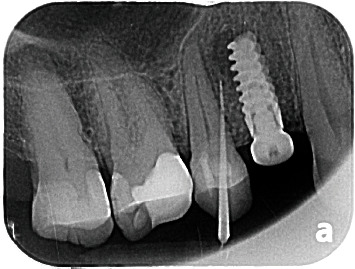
Radiographic presentation of the implant periapical lesion.
